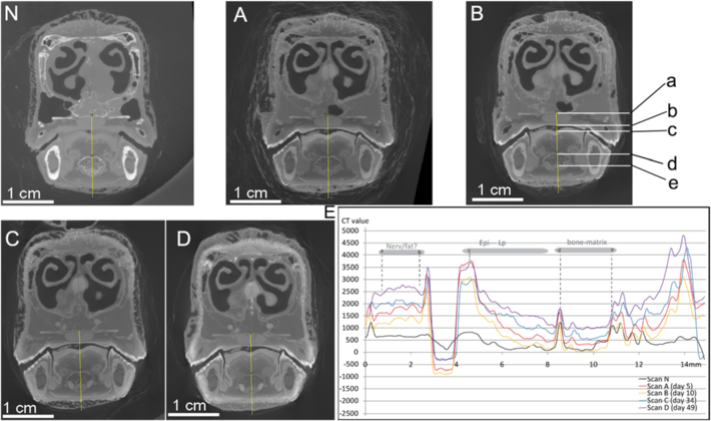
The coronal section of CT images crossing the nasal capsule and the middle region of the tongue (n, a-d) with CT values plots for targeted tissues selected (E). n, a, b, c, and d correspond to the five scans described as in Fig. 1; the same color regime was used for the CT plots as well. Distinct CT values with tissue identification were labeled and correlated between the plots (e) and positions on the line (b: *a*-*e*). Anatomical abbreviations: Epi, epidermis; Lp, lamina propria; Nerv/fat?, nerve or fat tissues
